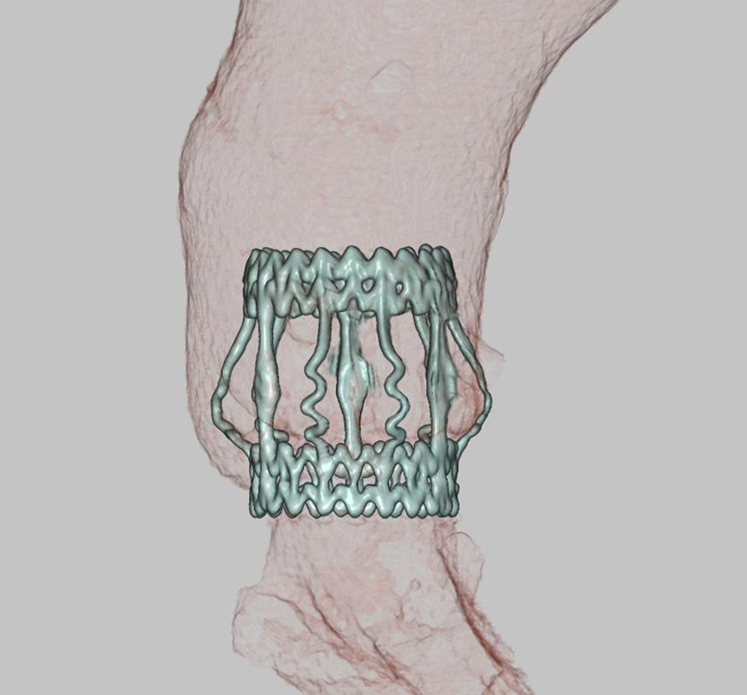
Postoperative 3-dimensional computed tomography demonstrating no evidence of an anastomotic pseudoaneurysm and confirming that the Perceval valve remained securely in position without evidence of migration.
